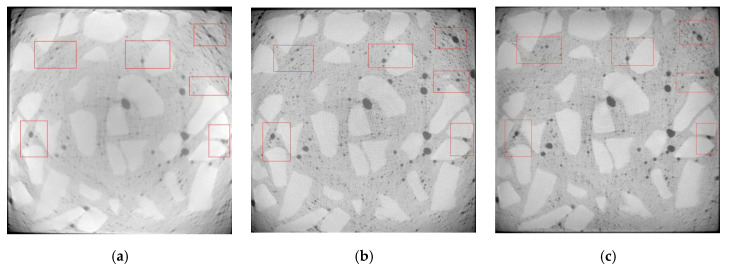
CT images of D5 under different dry and wet cycles of sulfate attack: (a) 0 dry–wet cycle; (b) 40 dry–wet cycles; (c) 80 dry–wet cycles.
